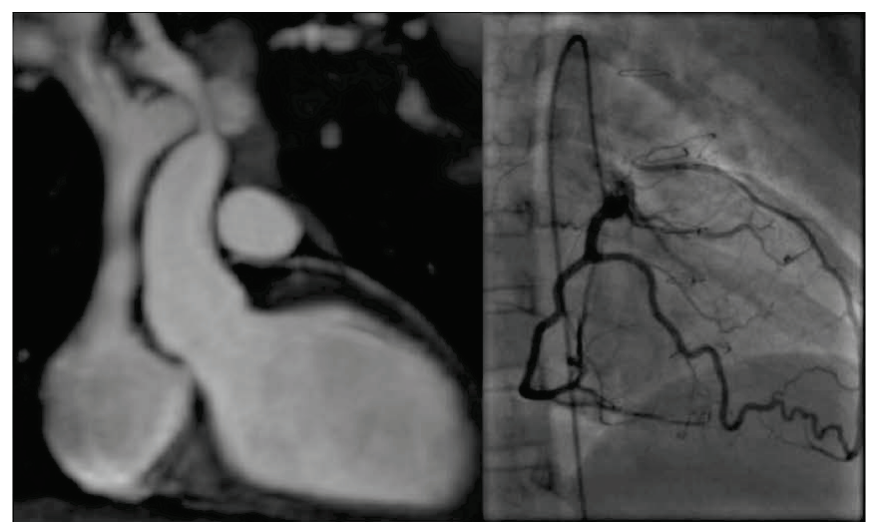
3D b-SSFP whole heart images, MRCA (left) shows no direct connection between the left coronary artery and the left aortic sinus of Valsalva. Findings confirmed on X-ray coronary angiography (right).
